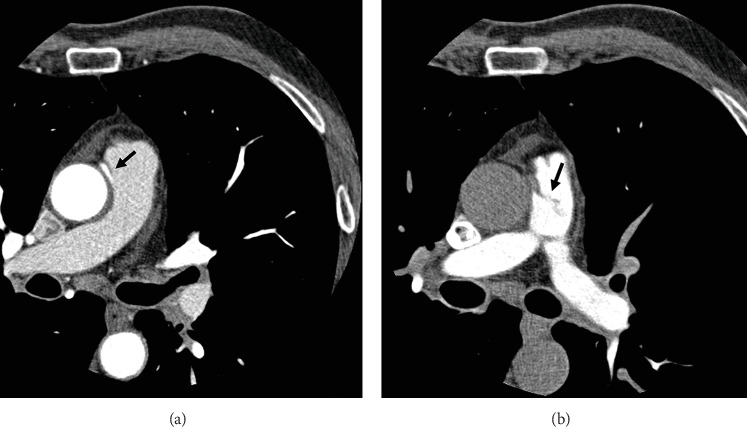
Computed tomography, axial plane arterial phase showing the anomalous origin of the right coronary artery from the pulmonary artery (arrow in a). In the venous phase, a retrograde filling toward the pulmonary artery is evident (arrow in b).
